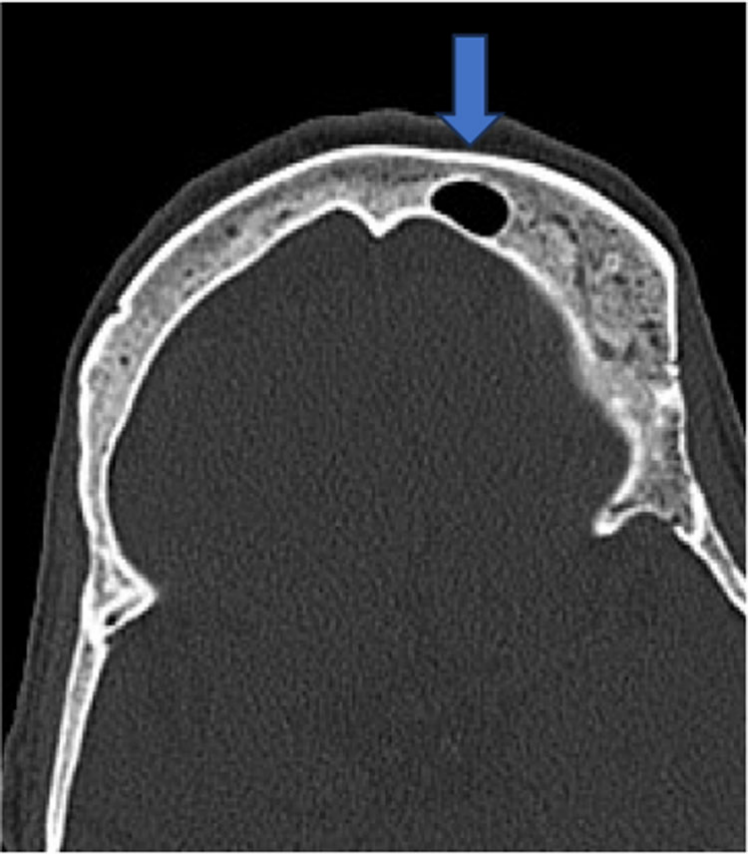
CT axial image shows unilateral frontal sinus.
